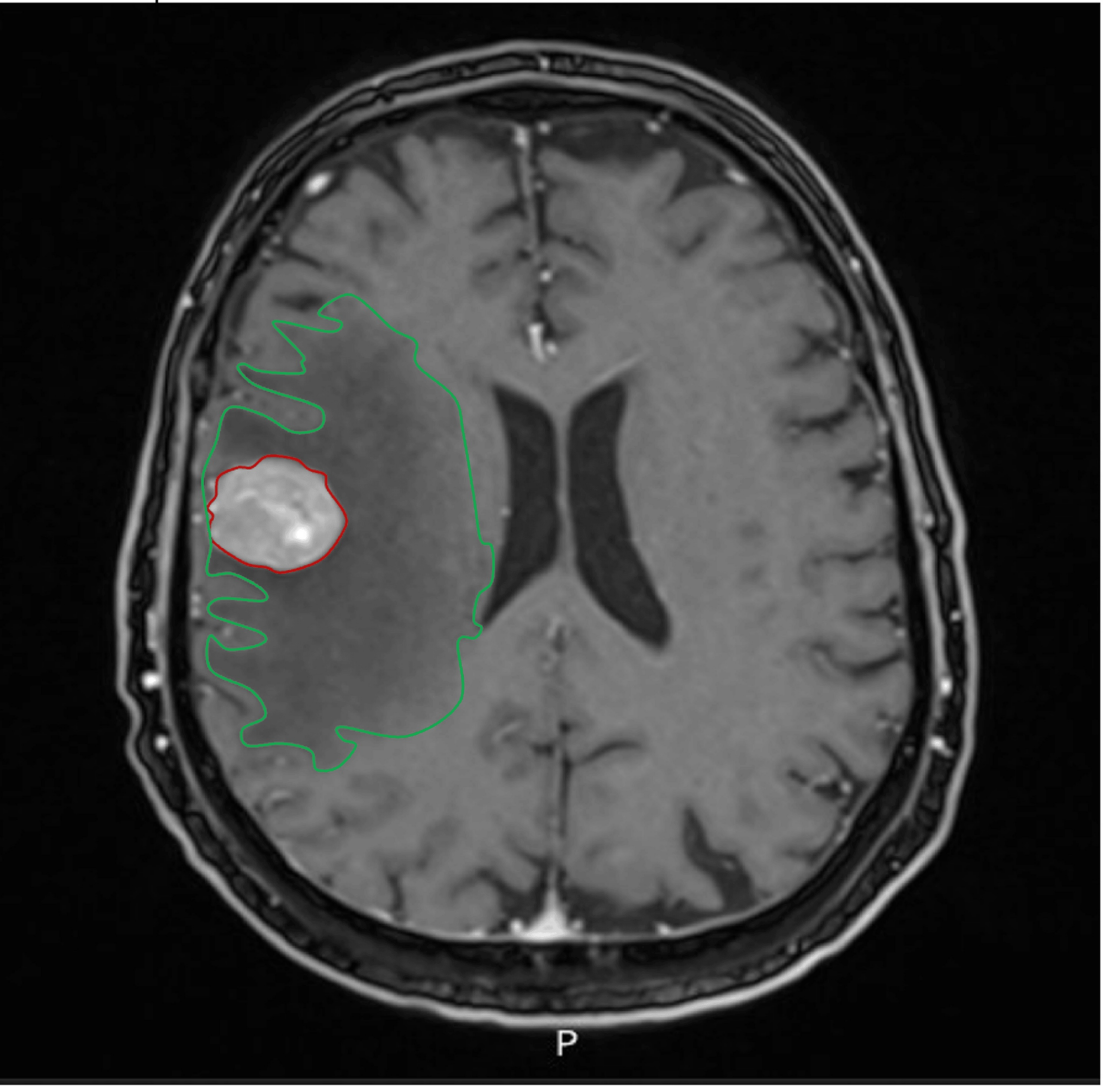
Post-gadolinium T1-weighted MRI image showing a heterogeneous enhancement. Post-gadolinium T1-weighted magnetic resonance imaging (MRI) image showing intense, nodular, and somewhat heterogeneous enhancement, particularly in the central part of the lesion, with a small non-enhancing area. The lesion (red circle) and the surrounding vasogenic edema (green circle) are indicated.
